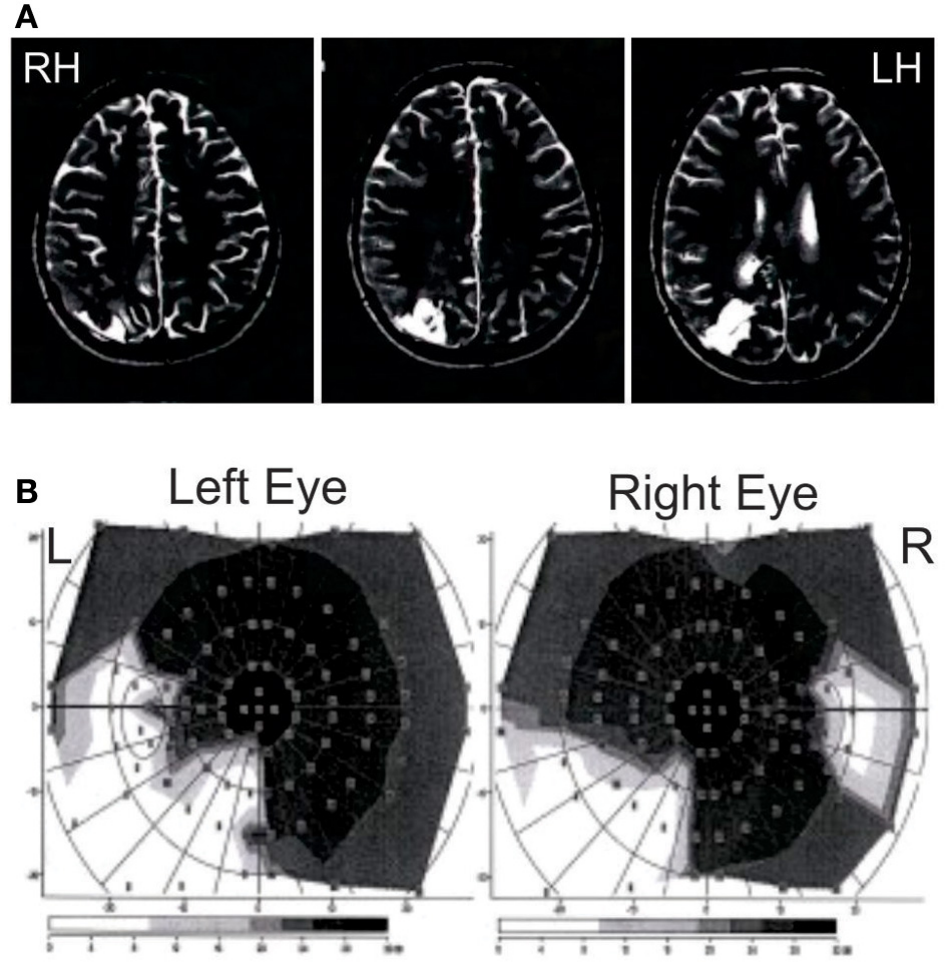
Patient JG MRI and visual perimetry. (A) MRI of patient JG. T2-weighted scans showing the damage after cyst removal (in white lower left) at the right parieto-occipital junction, including the caudal part of the intra-parietal sulcus and extending subcortically to the right lateral ventricle through the white matter. Scans are shown in radiological convention. (B) Goldman perimetry showing patient's quadrantanopia in the lower left visual field. She also showed somewhat large blind spots in both eyes.
